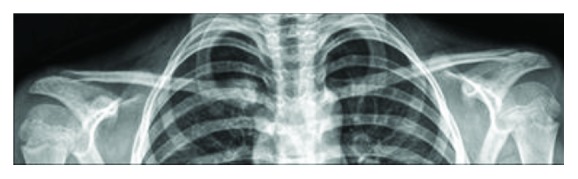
Preoperative anteroposterior radiographs revealing an asymmetric position of the medial clavicle on the right side.
